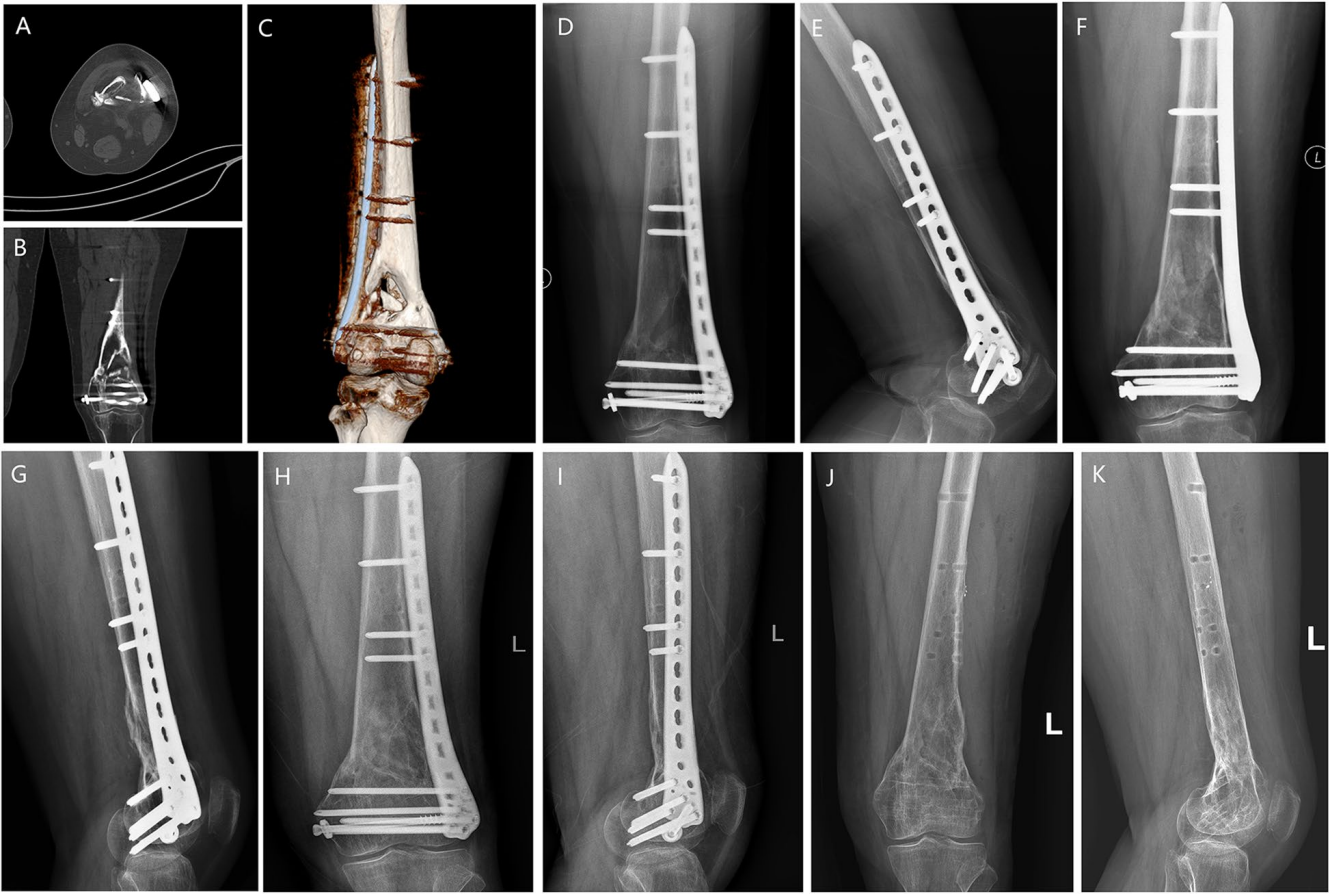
The CT scans (A, B, C) and the preoperative X-ray films (D, E) identified the non-union of femoral supracondyle. The Judet decortication technique was used to expose the non-union sites, and the paste comprised of DMB, PRP and allograft was implanted to fill the bone defect, covered with allogenous cortical bone around. Postoperative anteroposterior (F) and lateral (G) position films of X-ray were taken 3 days after surgery. The follow-up radiographic image showed bony union was achieved at 6 months (H, I). The hardware was removed 16 months postoperatively (J, K)
